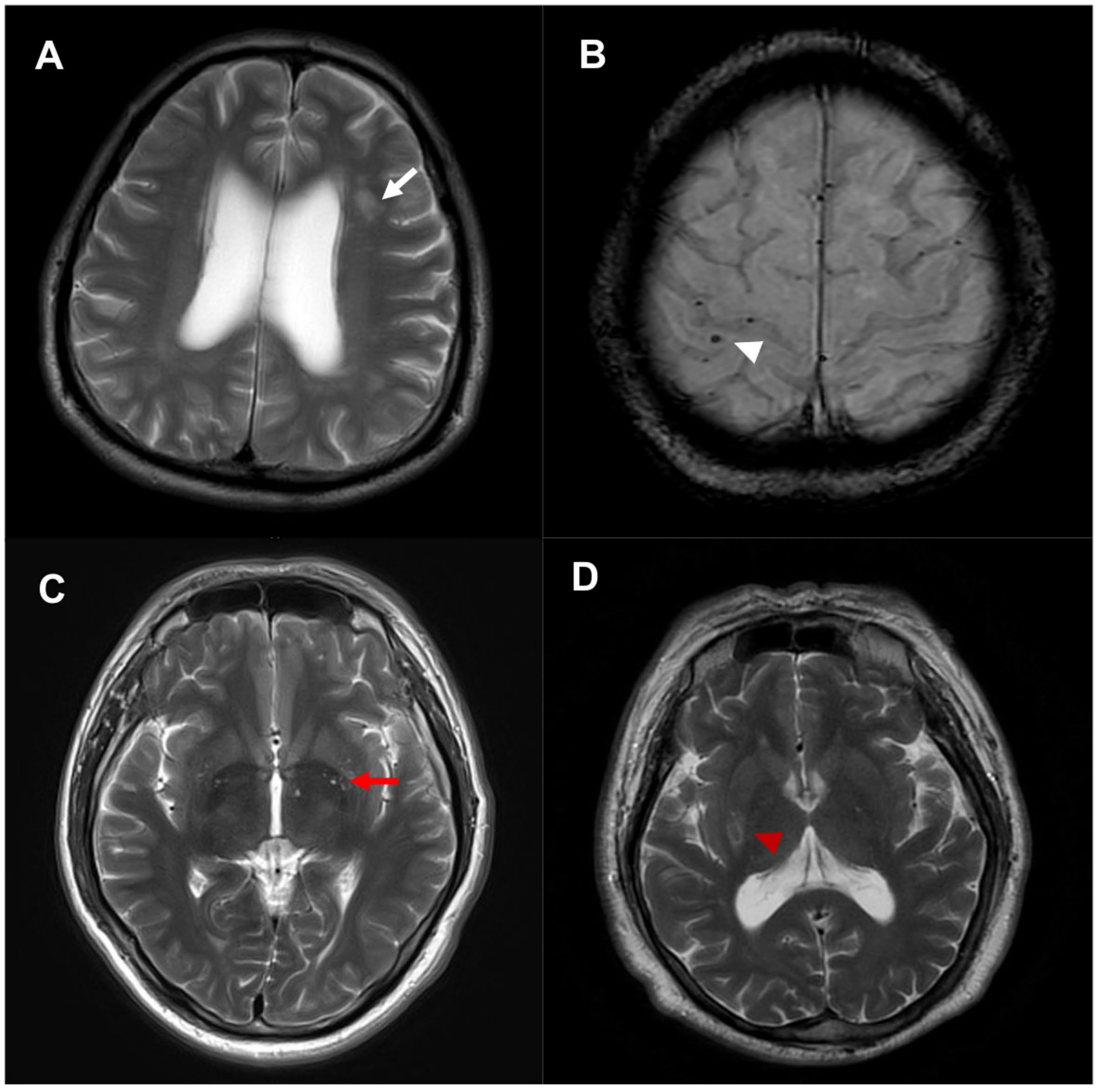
Cerebral small vessel diseases in brain MRI images: (A) White matter hyperintensity (white arrow) in T2-weighted image. (B) Cerebral microbleed (white arrowhead) in SWI image. (C) Perivascular spaces (red arrow) in T2-weighted image. (D) Lacunar infarction (red arrowhead) in T2-weighted image. MRI: magnetic resonance imaging; SWI: susceptibility-weighted imaging.
